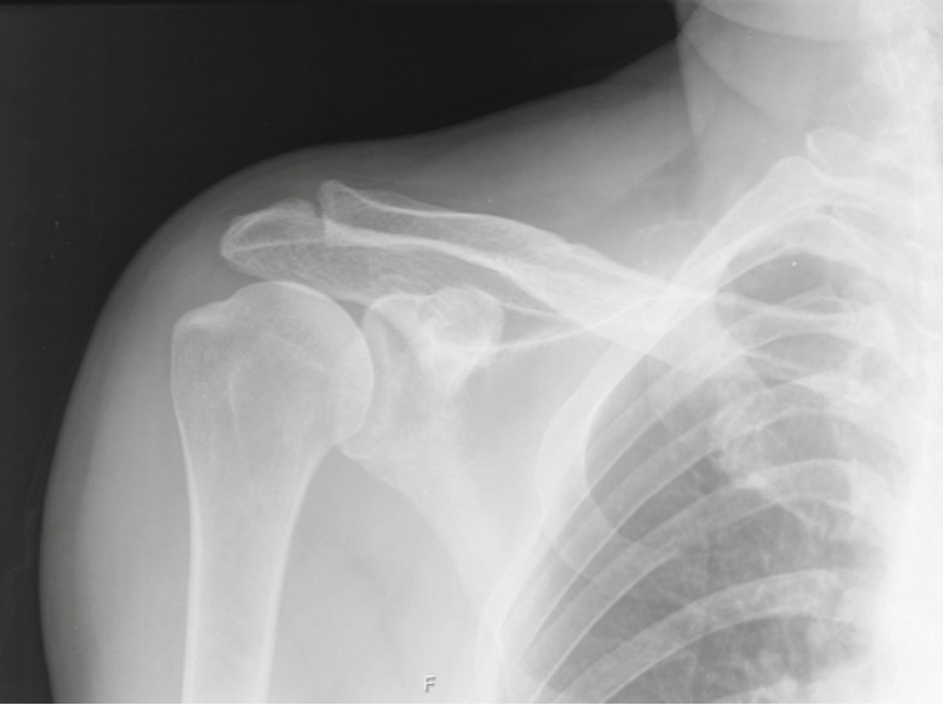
Anteroposterior radiograph of the right shoulder. The radiograph is normal.
